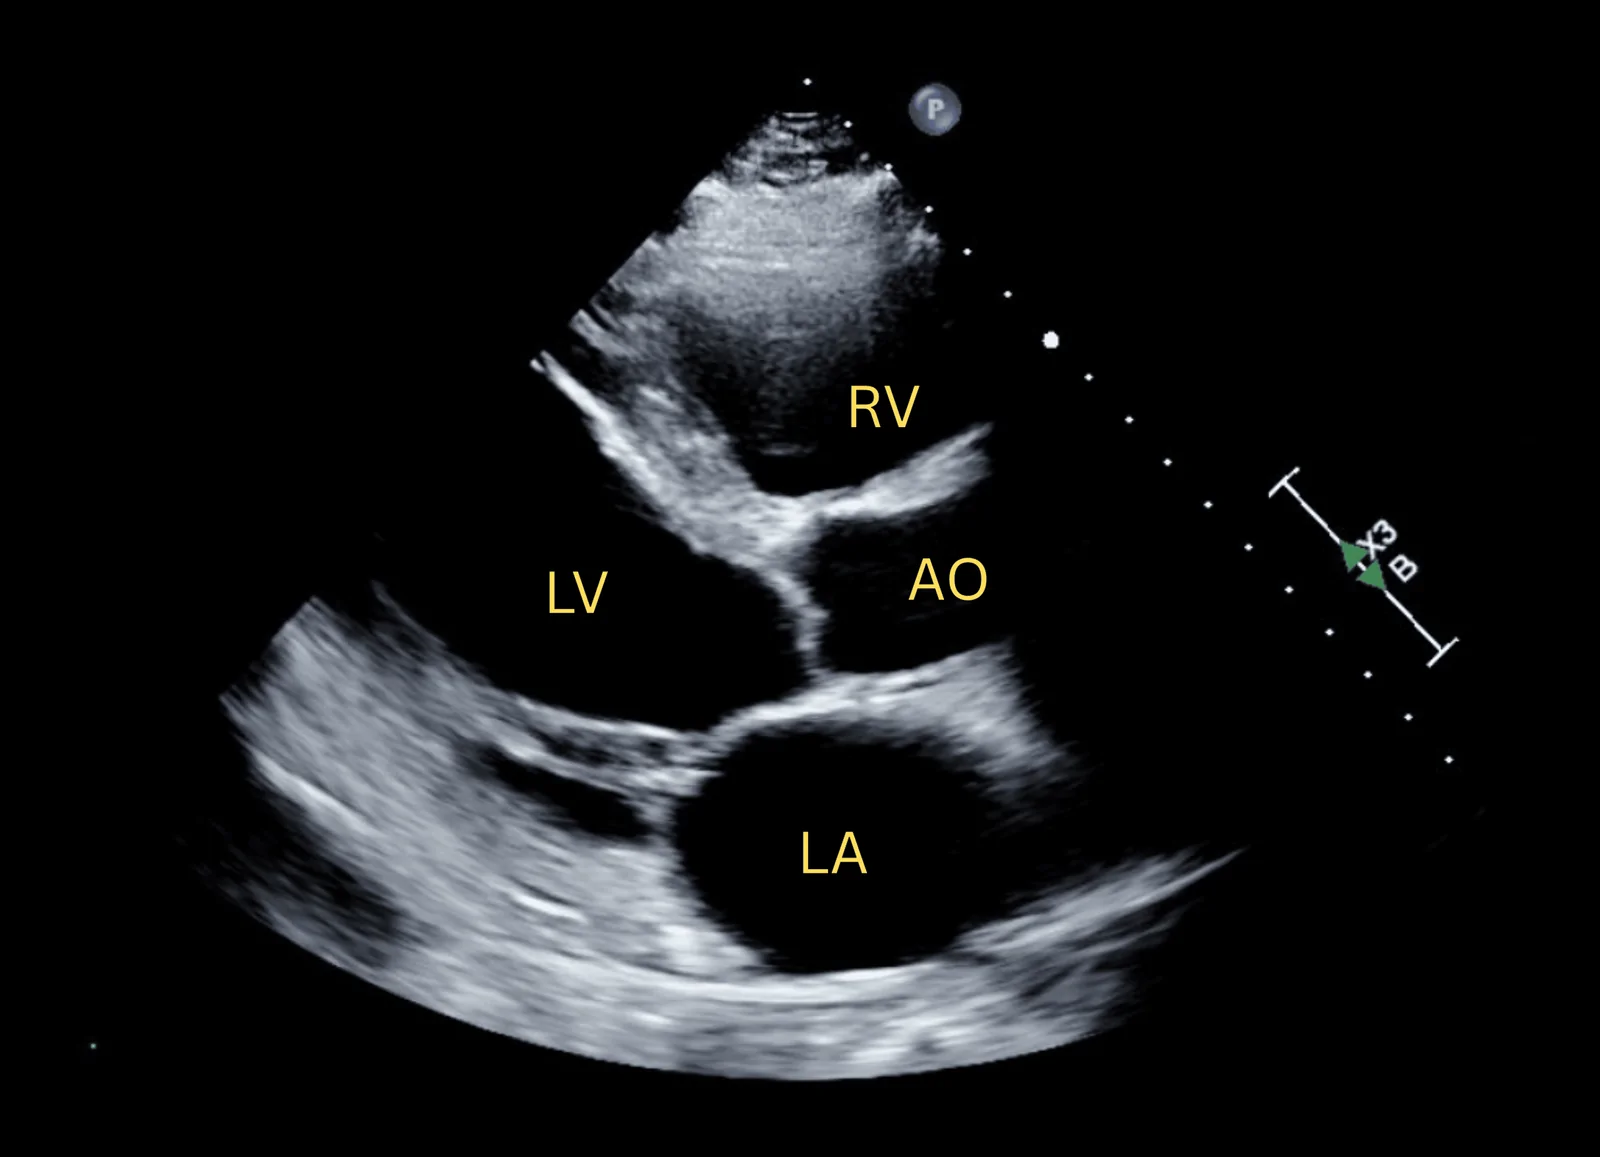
TTE demonstrating normal cardiac structure and function with preserved left ventricular ejection fraction (60-65%) and no significant valvular abnormalities. TTE: transthoracic echocardiogram; RV: right ventricle; LV: left ventricle; AO: aorta; LA: left atrium
